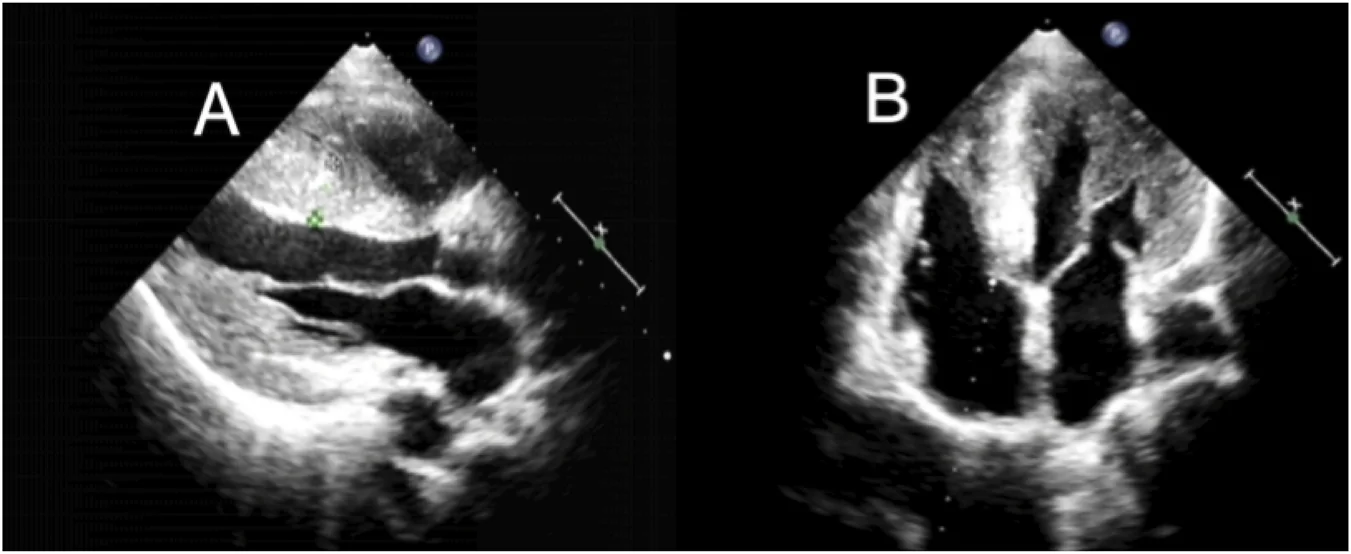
Transthoracic echocardiography (TTE) of the proband. Notes: Echocardiography reveals significant and asymmetric left ventricular hypertrophy, as demonstrated in the left ventricular short-axis (A) and four-chamber views (B). The maximal wall thickness is 18 mm at the interventricular septum and 18 mm at the posterior wall.
